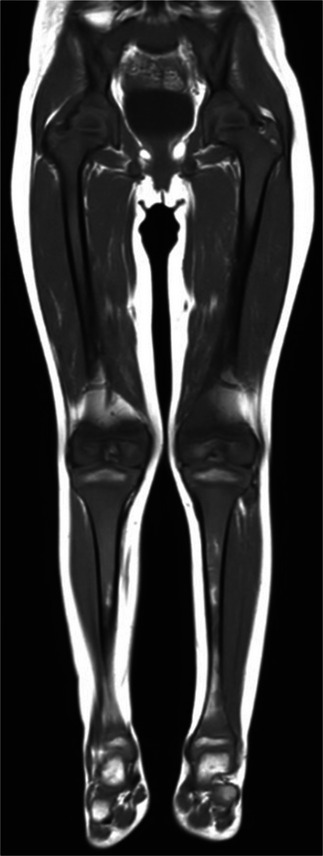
Leukemia. A 4-year-old girl with acute lymphocytic leukemia who presented to the emergency department with limp and fever. Coronal T1-weighted image of both lower extremities shows a diffuse marrow-replacing process with low signal intensity on the T1-weighted image throughout the bone marrow of the pelvis and the bones of both lower extremities. There was an increased marrow signal on a corresponding STIR image (not shown)
